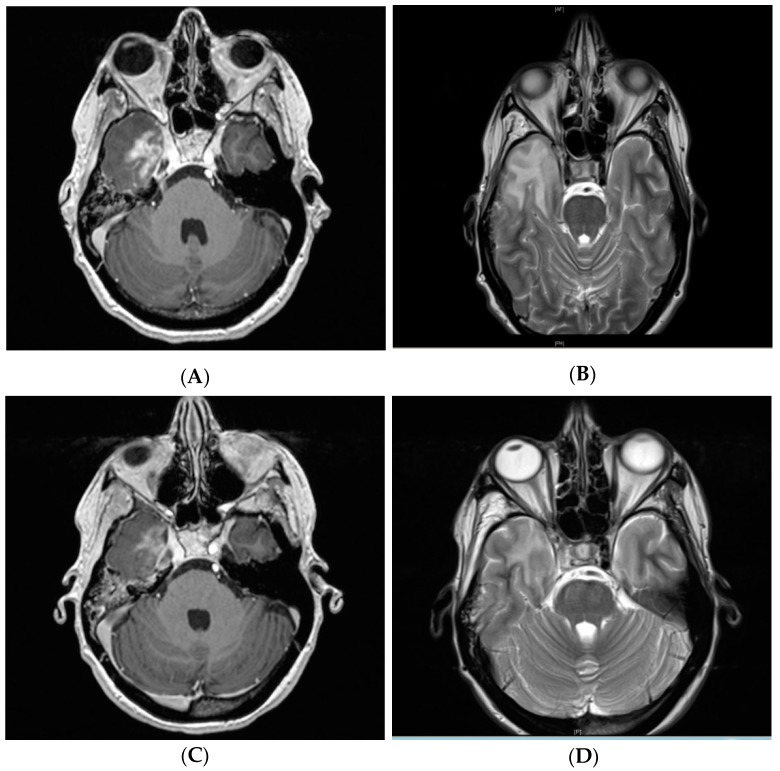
T1-weighted contrast-enhancement (A) and T2 alteration (B) post-treatment follow-up MR imaging of the same patient from Figure 2 showing grade III symptomatic CNSN in the right temporal lobe 11.9 months after treatment. Radiographic response after intravenous bevacizumab 7.5 mg/kg administered at two-week intervals for four treatments with residual T1-weighted contrast-enhancement (C) and T2 hyperintensity (D). The patient also responded clinically with swift recovery from double vision, vertigo, and headaches.
